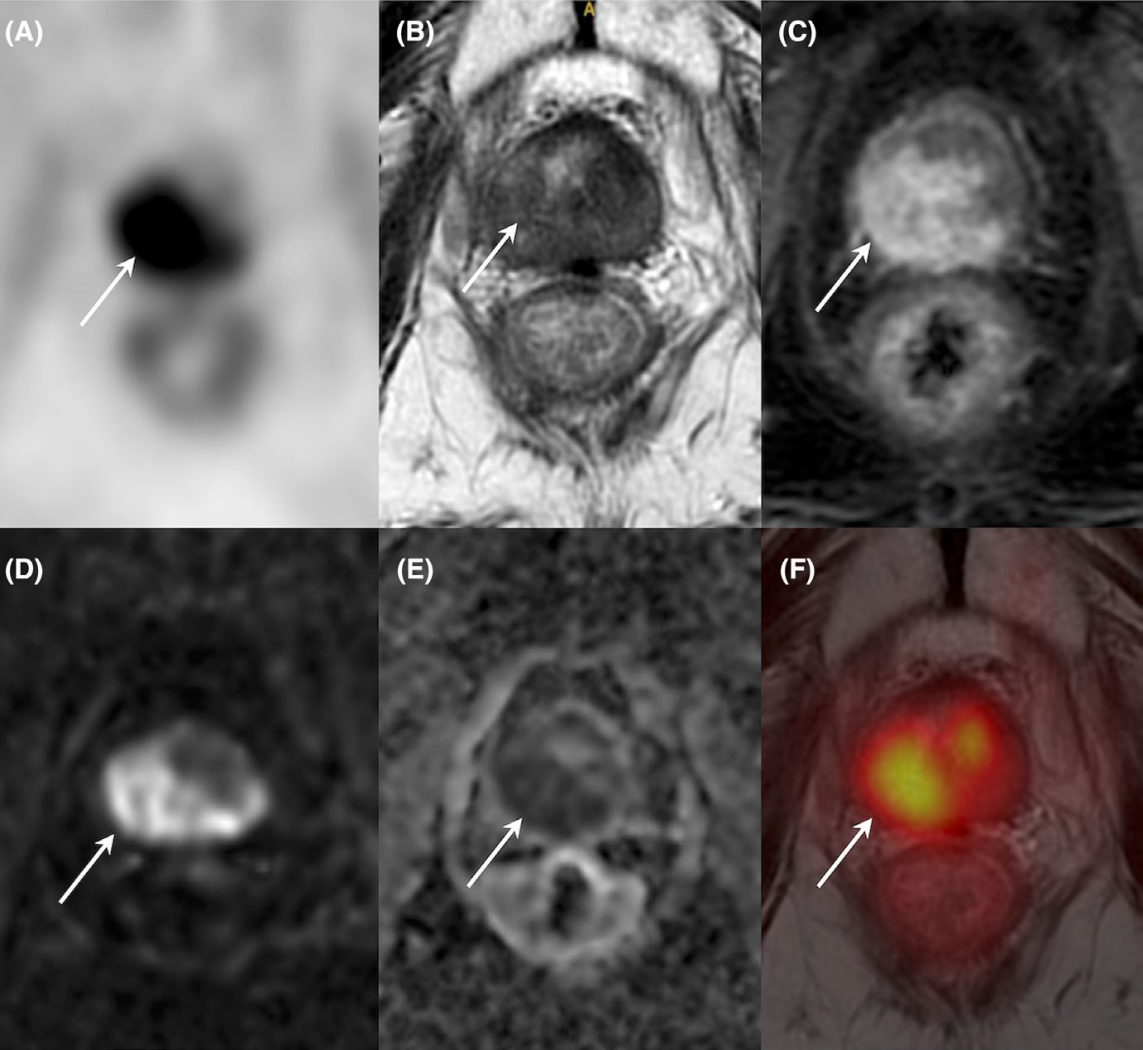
Typical results of [^68^Ga]PSMA PET/MRI imaging in a patient with prostate cancer (primary staging) developed in the peripheral zone of the right lobe and capsular infiltration (axial PET (A), T2‐weighted MRI (B), contrast‐enhanced MRI (C), Diffusion Weighted (DWI) MRI (D), Apparent Diffusion Coefficient (ADC) MRI (E), PET/MRI hybrid imaging (F)). Tumour appears as a 15 mm hypointense T2‐weighted lesion with high contrast enhancement, diffusion restriction, and intense [^68^Ga]PSMA uptake (arrows).
